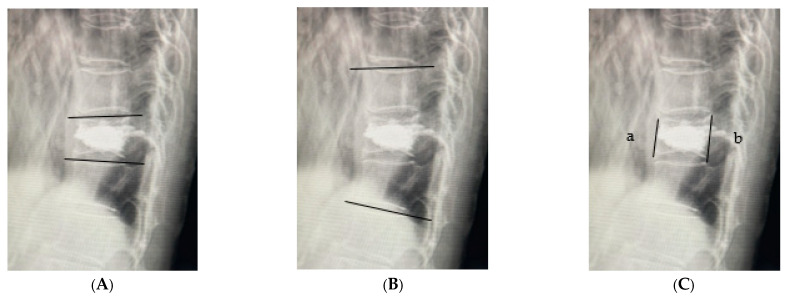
Measurement of plain lateral radiography. (A) Vertebral kyphosis angle; (B) Local kyphosis angle; (C) Vertebral wedge ratio (a/b × 100).
